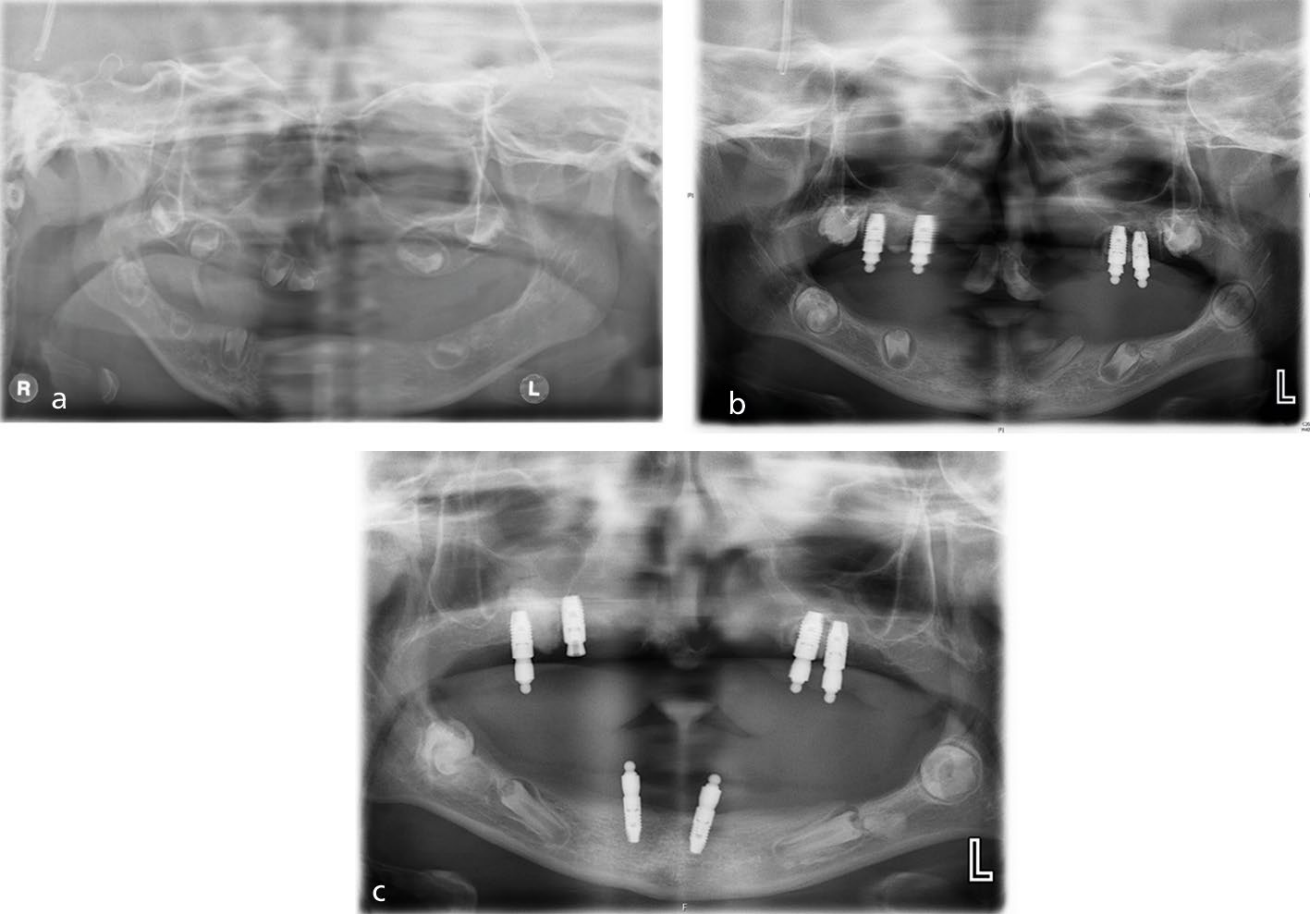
a, b, c) Panoramic radiographs taken in 2012, 2015 and 2018, of a child with ectodermal dysplasia and bilateral cleft lip and palate, demonstrating the progressive use of dental implants in the developing patient. Reproduced with permission from L. Clarke *et al.*, 'Britain's youngest implant patients? - A Case Series of implant treatment in children with ectodermal dysplasia', *Oral Surgery*, 2020, John Wiley & Sons^13^
